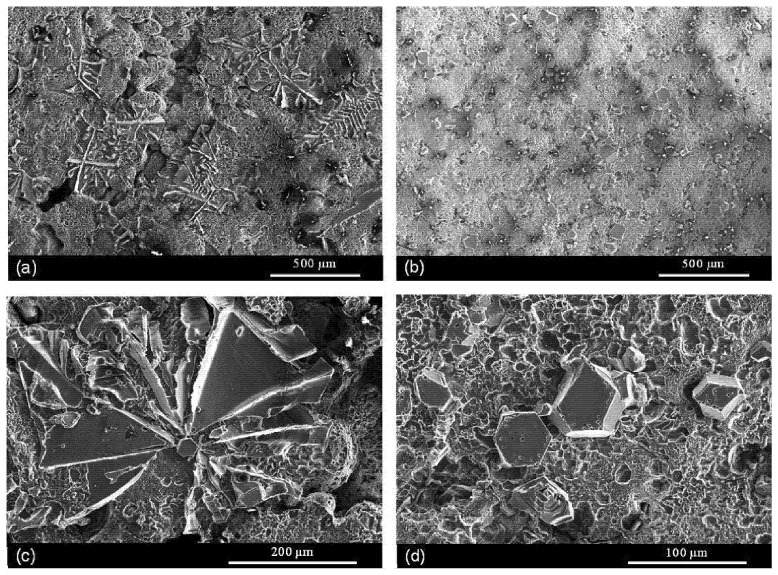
Deep-etched microstructure of the α-Al_15_(Fe, Mn)_3_Si_2_ intermetallic formed: (a,c) without UST and (b,d) with ultrasonication. Reproduced from [89] with permission from Elsevier, 2022.
